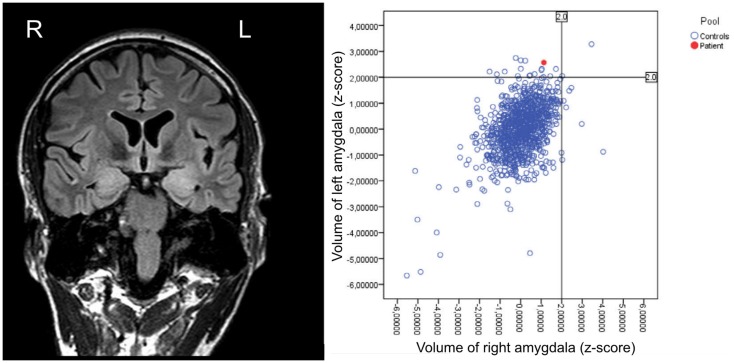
Structural MRI (performed in February 2012) showing bilateral swelling with signal alterations of the temporomesial structures more prominent on the left hemisphere, and respective volumetric results revealing enlargement of the left amygdala as compared to healthy controls.
